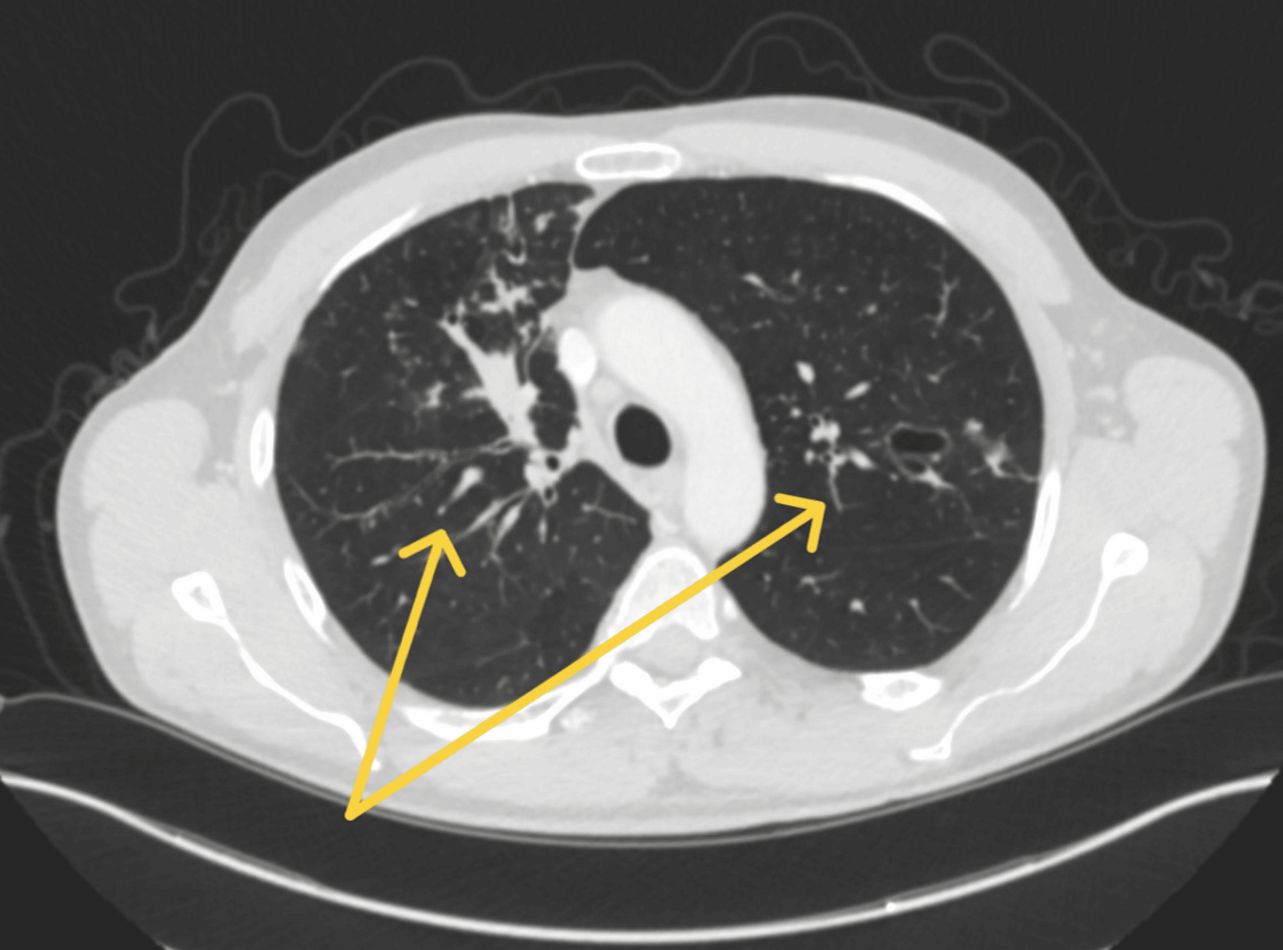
Follow-Up CT Chest Yellow arrows: mild improvement in the overall density of the chronic fungal infection in the right upper lobe. Multiple nodular opacities in the left upper and lower lobes remained stable.
